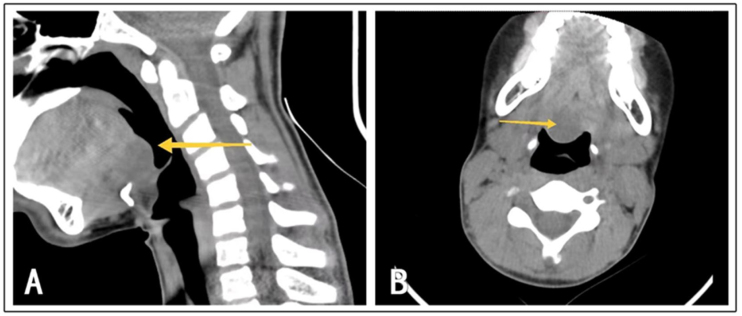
(A) Case 2: Location of the intraoral thyroglossal duct cyst as shown on the CT sagittal plane. (B) Case 2: Location of the intraoral thyroglossal duct cyst as shown on the CT axial plane.
